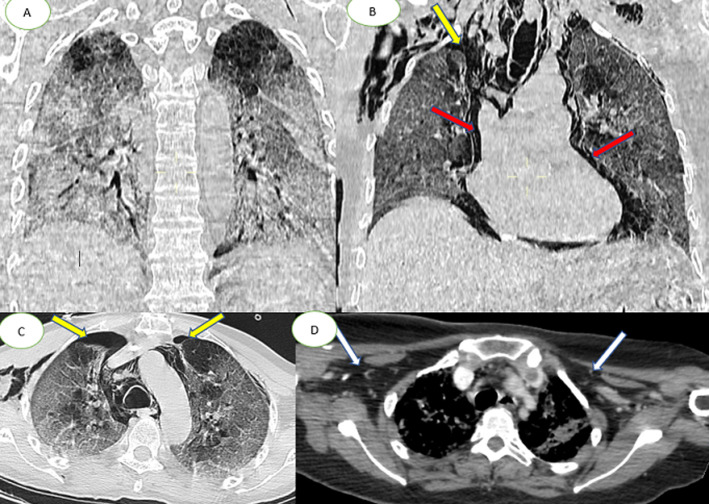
chest CT scan in coronal (A, B) axial (C, D) and lung parenchymal windows showing subpleural ground-glass opacities associated with multiple areas of consolidation as well as the presence of bilateral pneumothorax, (yellow arrows) pneumomediastinum, (red arrows); extensive bilateral subcutaneous emphysema (white arrows)
